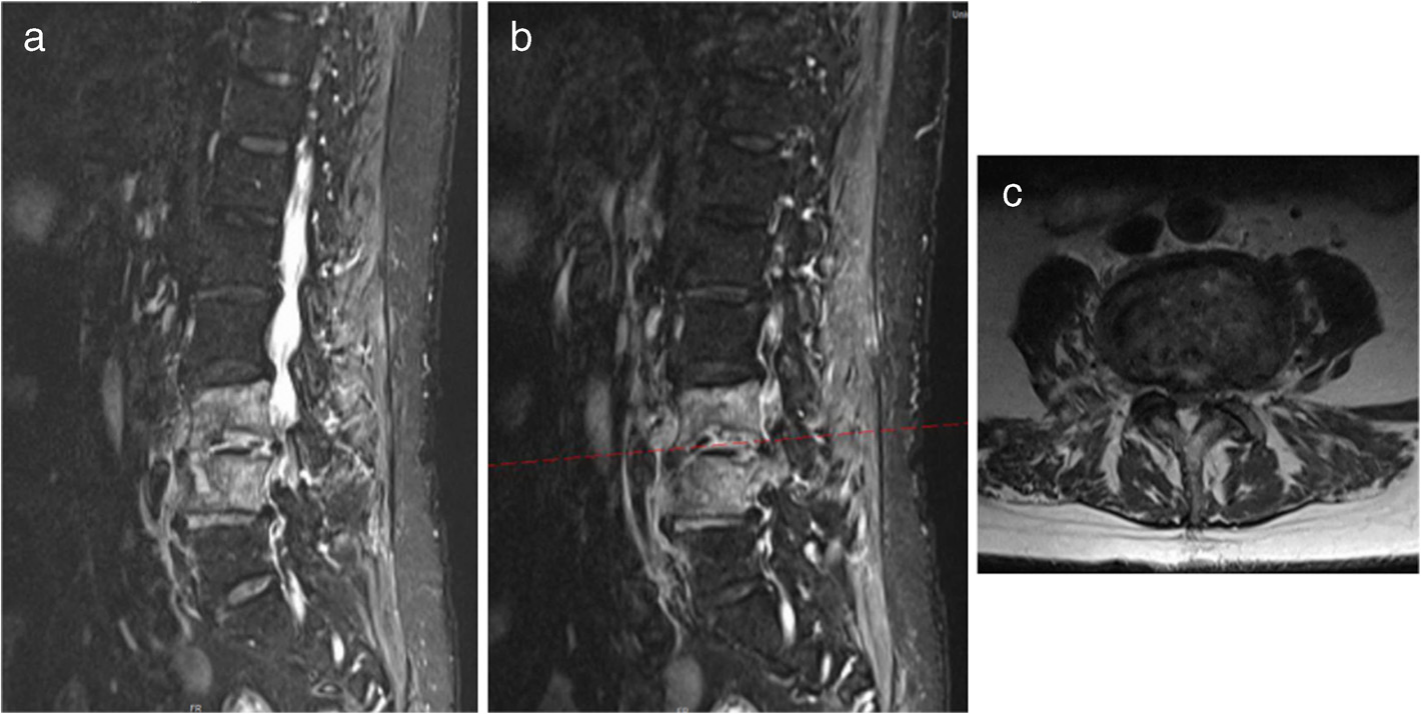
Examplary case of a 77 year old female patient presenting with immobilizing low back pain (VAS 10/10), instability, secondary kyphosis and an elevated CRP level of 119 mg/l. Pre-operative sagittal MR T2-weighted (a) and STIR (b) images demonstrate significant signal enhancements with arrosions of the bony endplates at the L3/4 level, along with a suspected infection of the L4/5 disc. The axial MR T2-weighted image of the L3/4 level (c) shows advanced degenerative changes of the facet joints with consecutive bilateral stenosis of the neuroforamen and spinal canal
